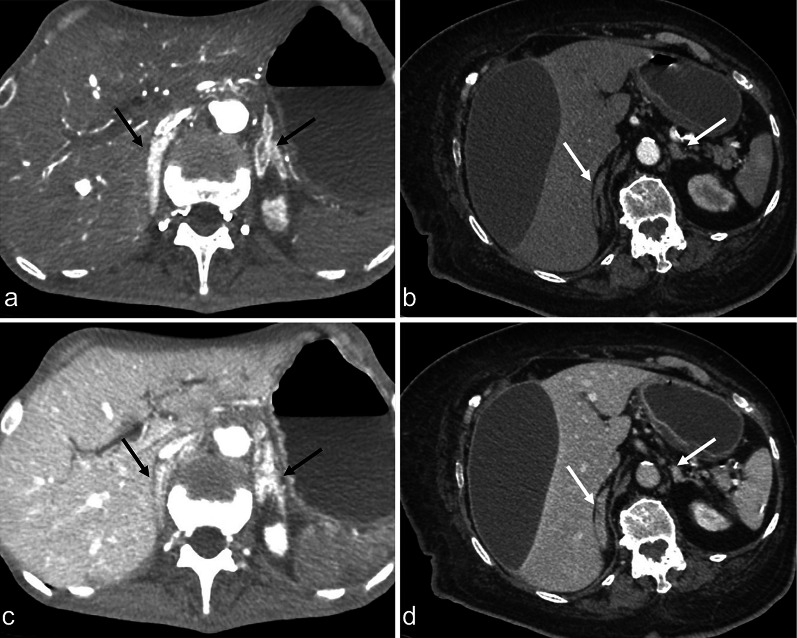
Contrast-enhanced CT images in the arterial phase from two different patients with septic shock (a and b) and the respective venous phases (c and d). Different patterns of adrenal gland hyperenhancement can be observed, characterised in one patient by a central zone with less intense enhancement relative to the peripheral zone (a, black arrows) and in the other patient by a central zone with heterogeneous enhancement and a mosaic-like appearance (b, white arrows). In both cases, the venous phase shows a homogeneous enhancement (c, black arrows; d, white arrows)
